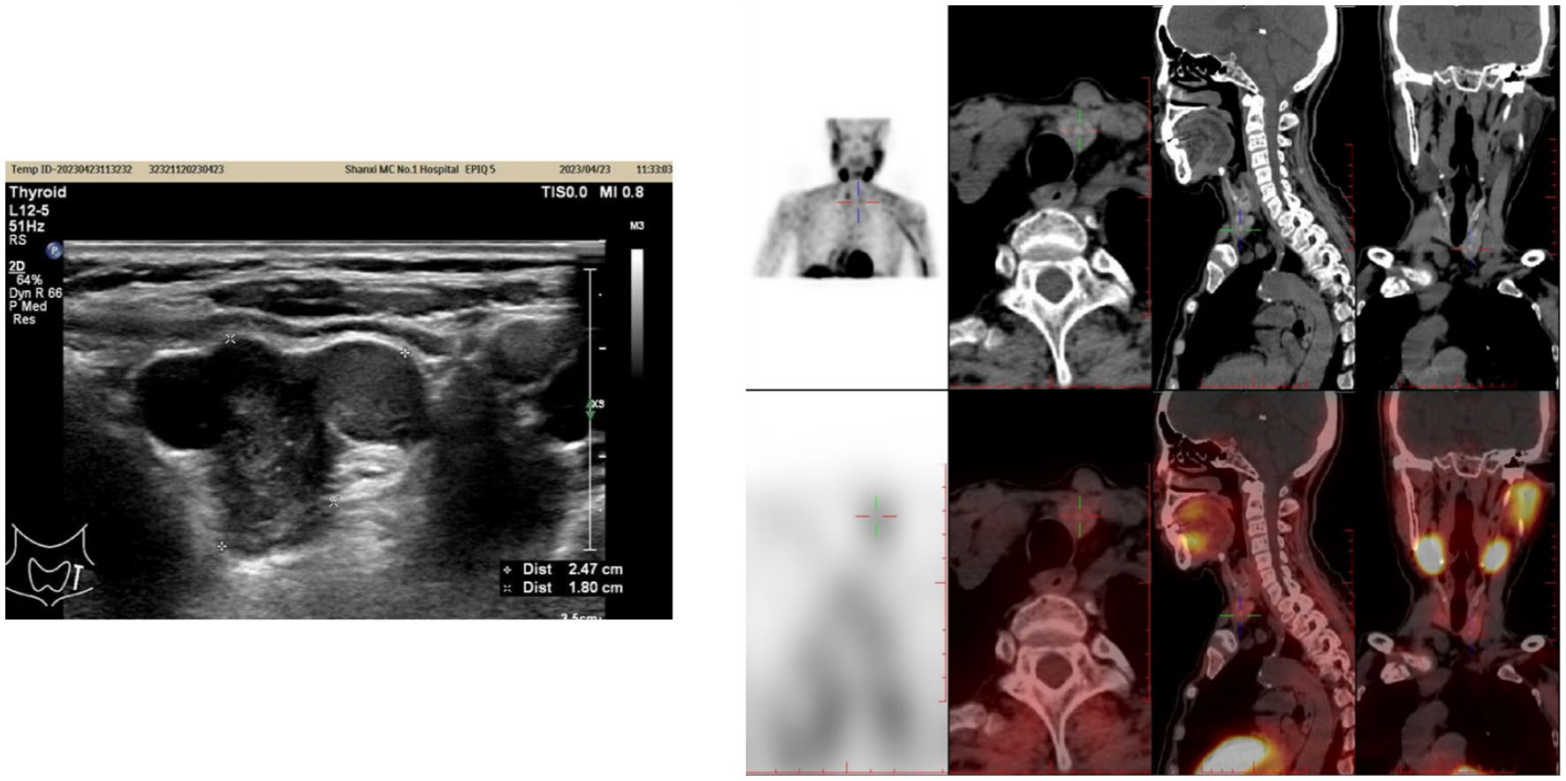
(A) Parathyroid color Doppler ultrasound image performed in April 2023. (B)
